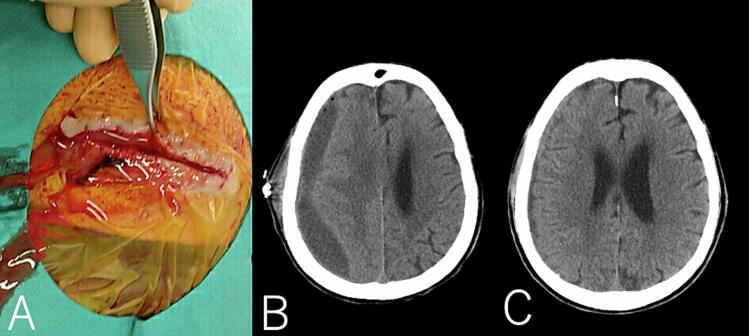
A: Pus was oozing from the wound. B: Head Computed Tomography (CT) reveal a recurrent abscess in the right epidural space. C: No recurrence was observed in the CT scan at the time of discharge.
